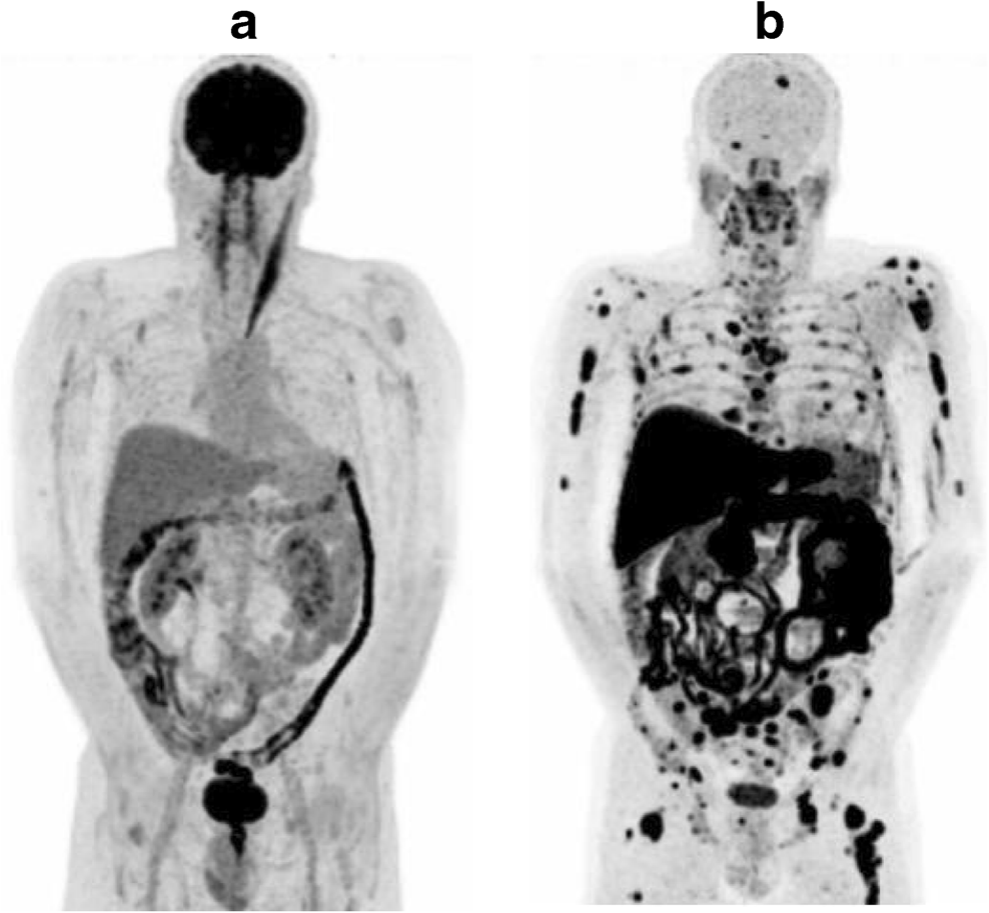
^18^F-FDG (a) ^11^C-methionine (b) PET/CT images in a patient with multiple myeloma. More lesions are shown by ^11^C-methionine PET/CT (b) than by ^18^F-FDG (a)
